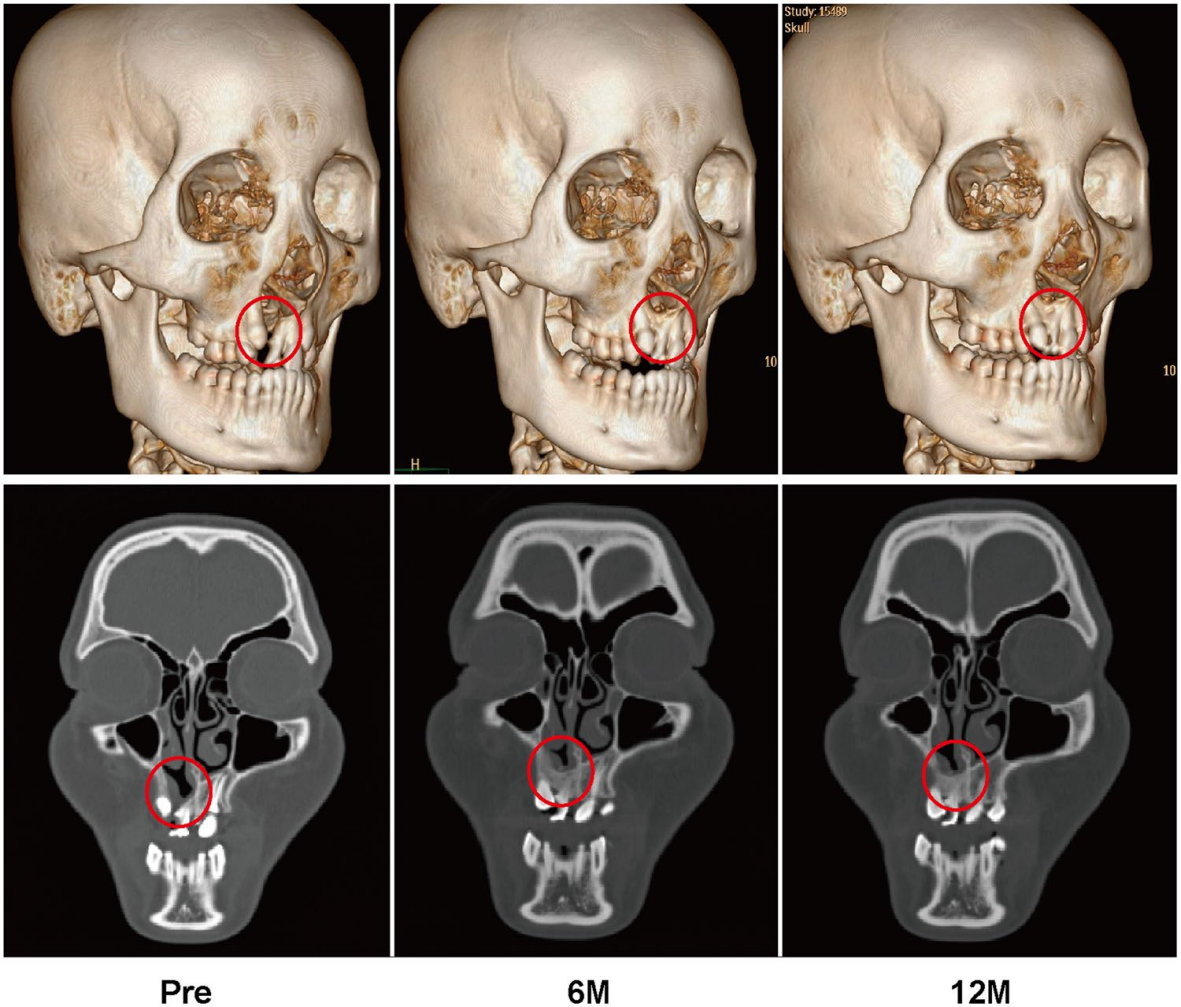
Typical three-dimensional and coronal computed tomographic images of a patient who underwent ICBG grafting. From left to right, images taken preoperatively and at 6 and 12 months postoperatively.
